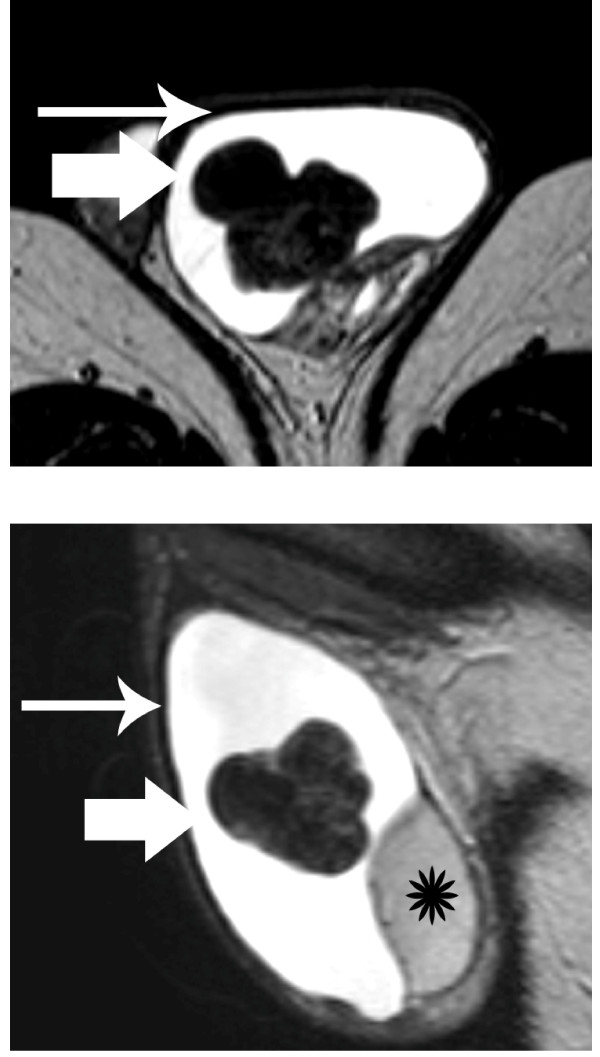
T2-weighted images (a) Transverse and (b) sagittal T2-weighted images show tumor heterogeneity. The mass (arrow) was mainly hypointense on T2-weighted images, a finding suggestive of the presence of fibrous tissue. Left hydrocele (long arrow). Normal left testis (asterisk).
